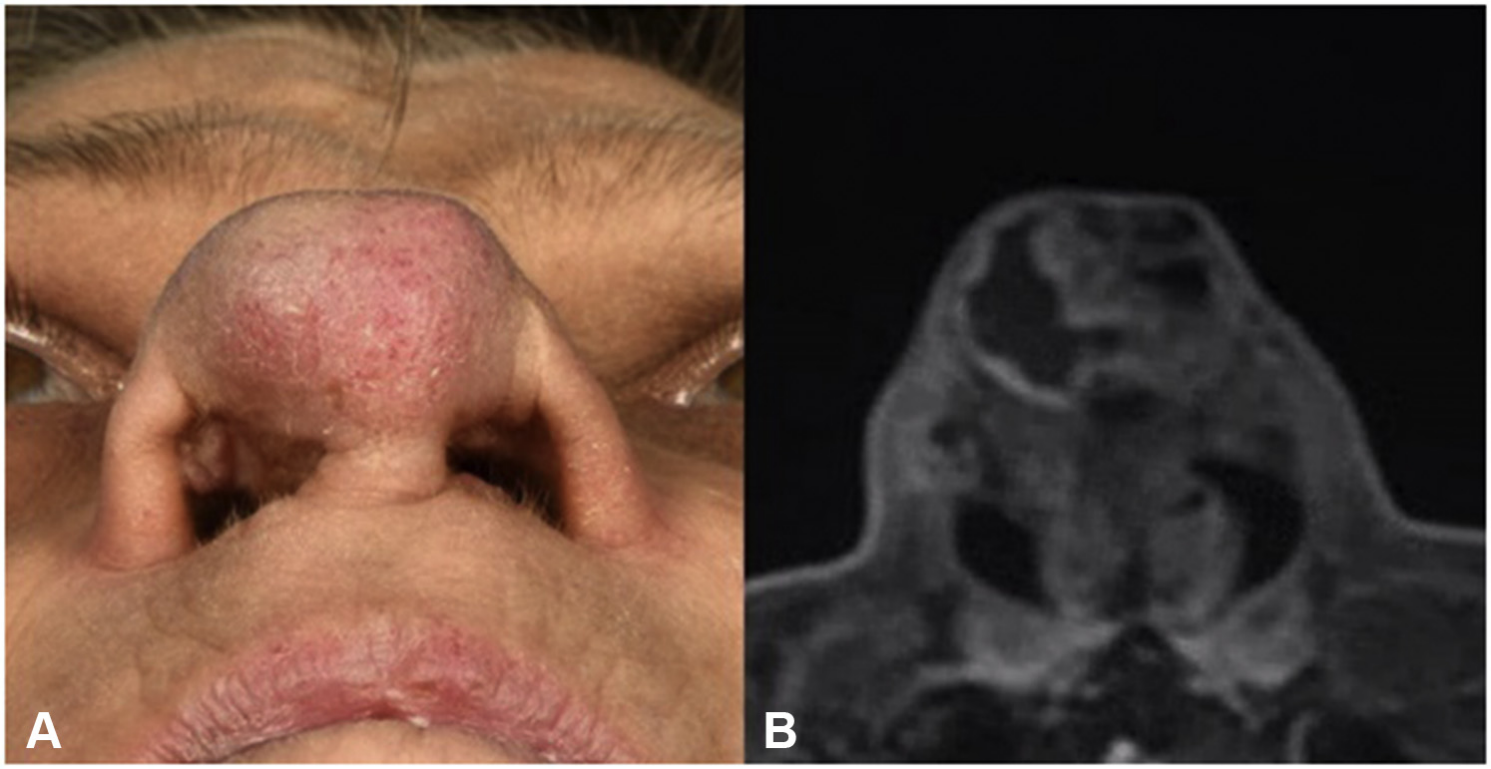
A, Basal view of the nasal tumor (*left*) and (B) the corresponding T1 MRI sequence (*right*). *MRI*, Magnetic resonance imaging.
